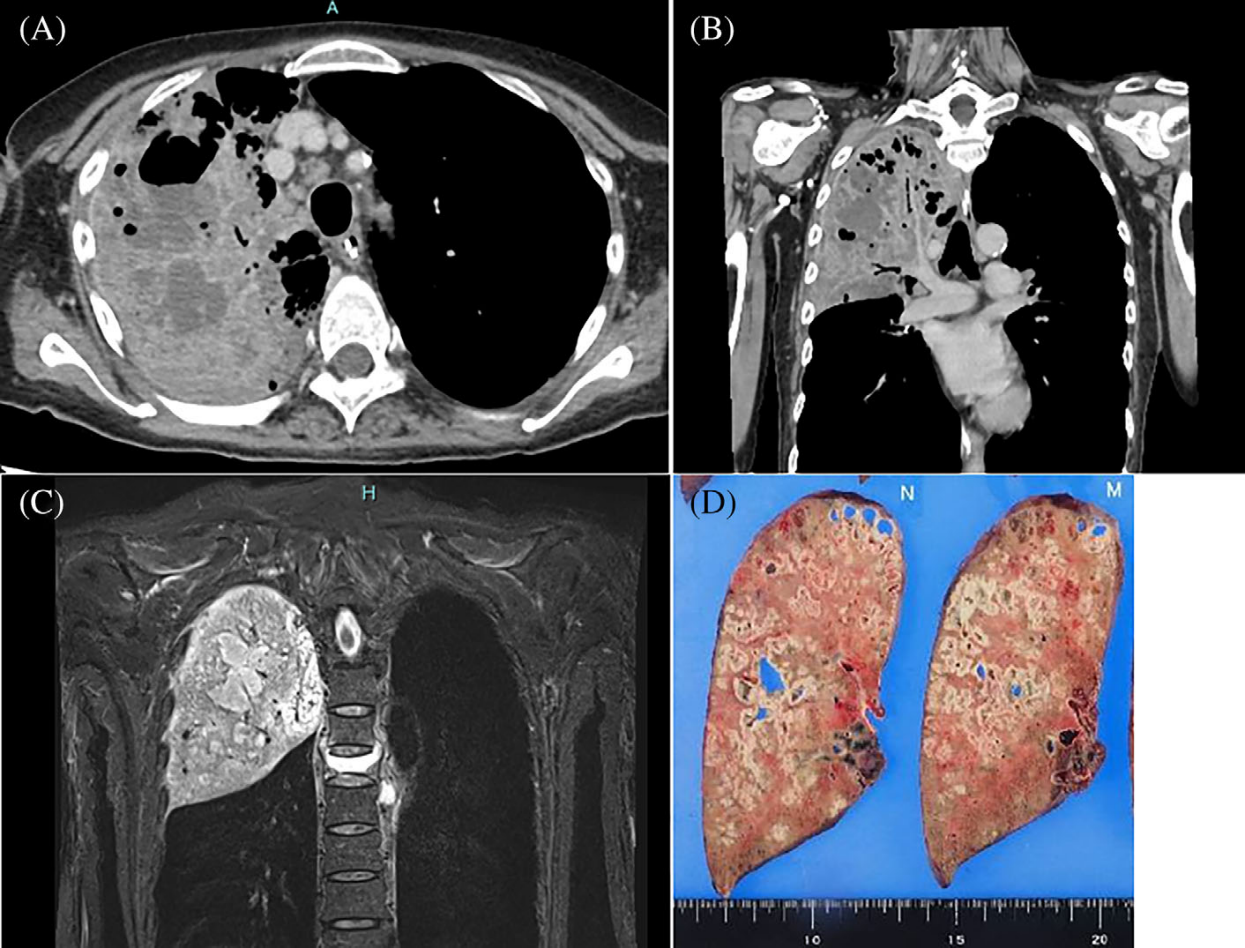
Multilocular lung abscess of the right upper lobe. Contrast‐enhanced computed tomography (A, axial; B, coronal) and magnetic resonance imaging (C; T2 weighted image) demonstrated the involvement of a multilocular lung abscess in her right upper lobe. The resected upper lobe showed the involvement of a multilocular lung abscess (D; macro picture).
